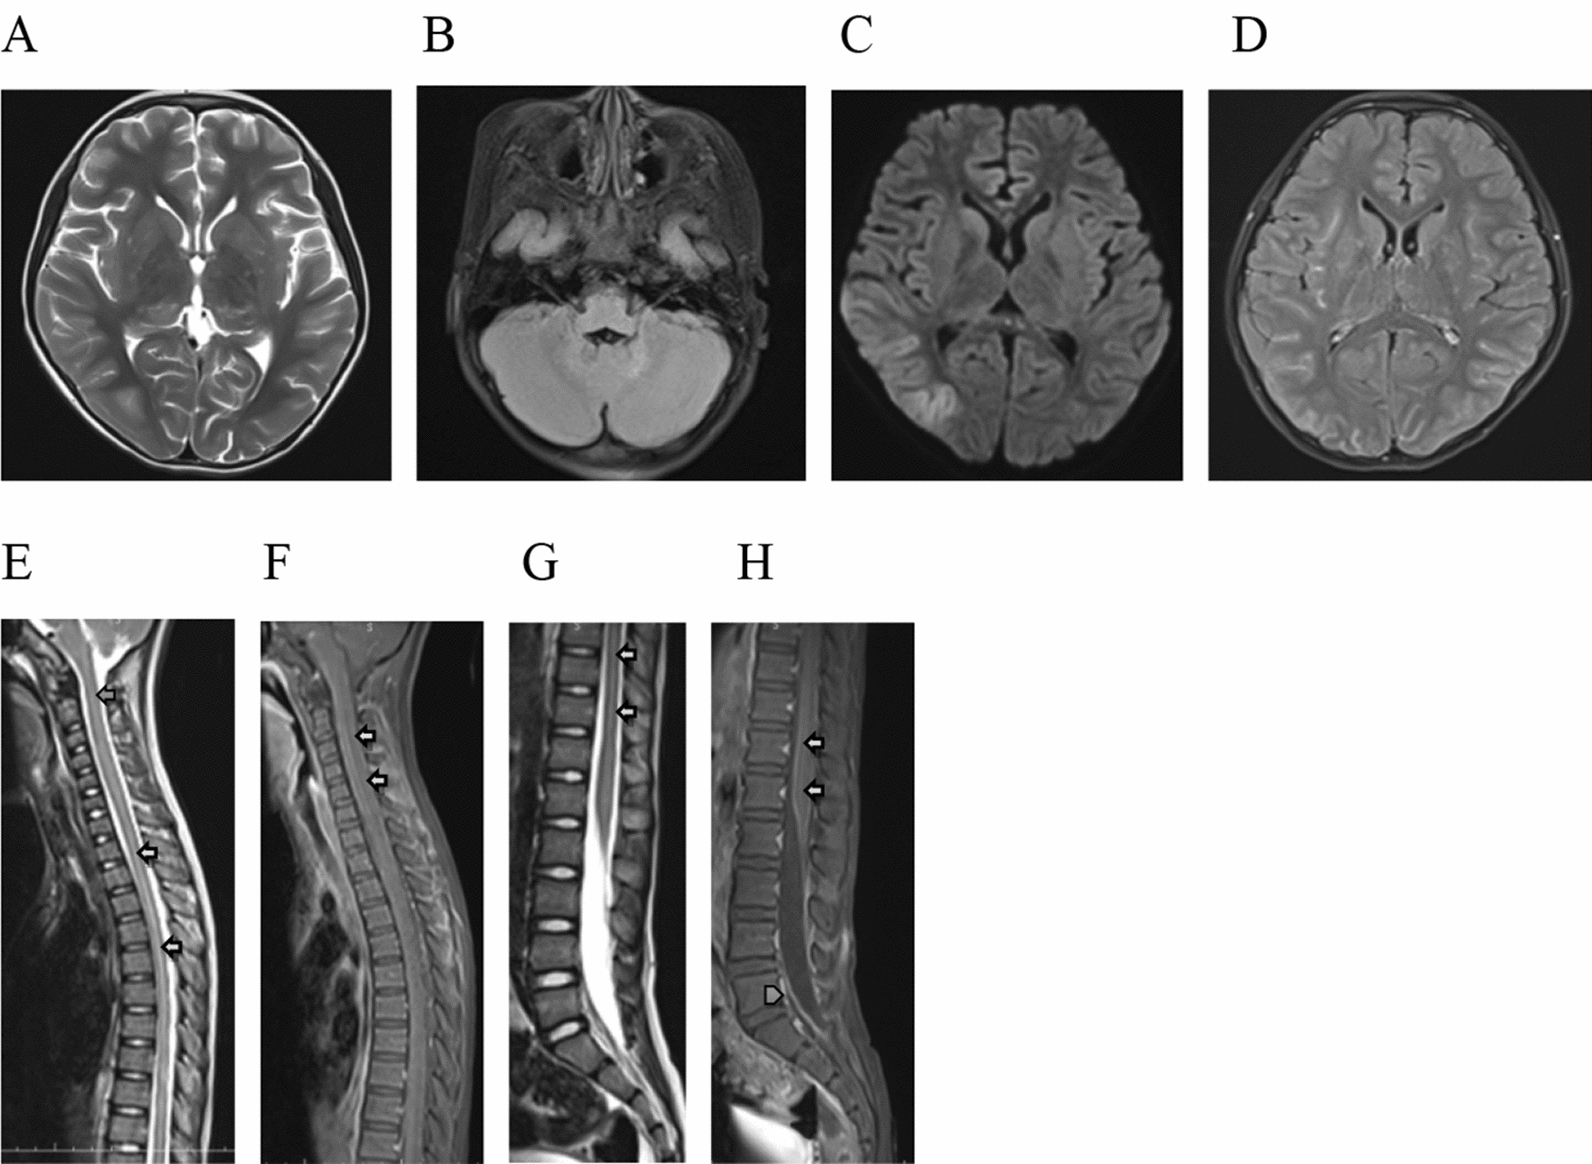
Imaging findings in pediatric patients with autoimmune GFAP astrocytopathy. Magnetic resonance imaging (MRI) showing abnormal hyperintensity lesions on T2-weighted and fluid-attenuated inversion recovery (FLAIR) images in basal ganglia, hypothalamus (A) and cerebellum (B). Diffusion-weighted sequences imaging (DWI) showed abnormal hyperintensity lesions in the callosum and cortical (C). Gadolinium-enhanced brain MRI (T2-FLAIR) showed enhancement leptomeninges (D). Spinal cord MRI showed longitudinally extensive spinal cord lesions (LESCLs) (arrows) (E, G) and slight enhancement (arrows), pia enhancement (arrowhead) (F, H)
